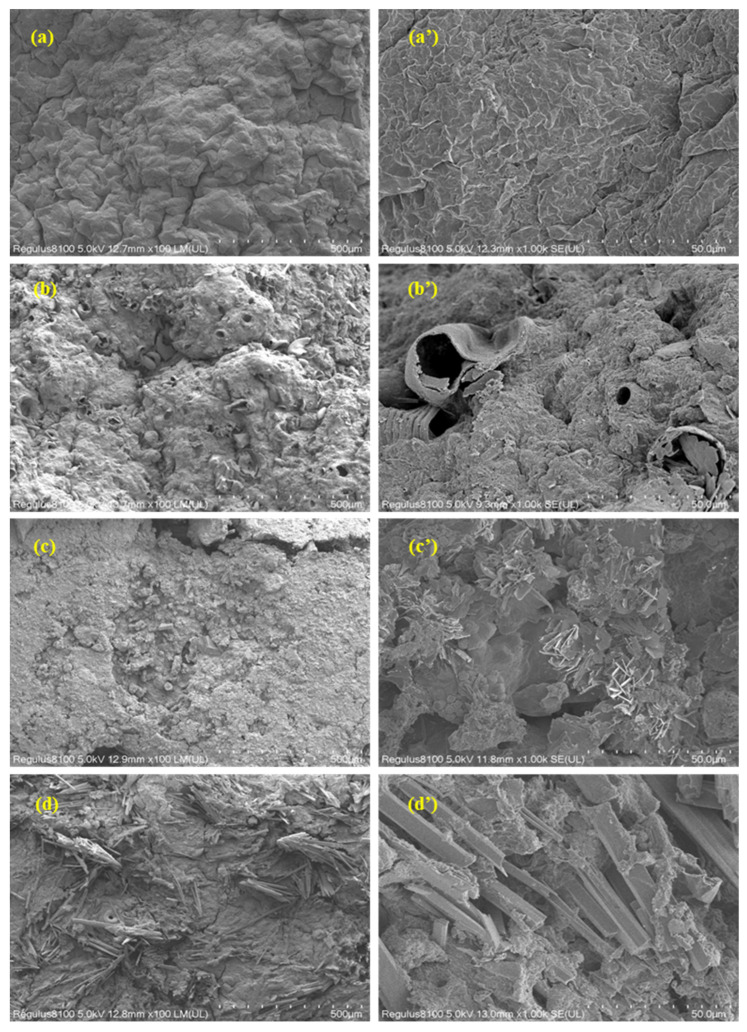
The SEM images of the filter cake after different conditionings: (a) raw sludge at the scale of 500 μm; (a’) raw sludge at the scale of 50 μm; (b) ultrasonic-pretreated sludge at the scale of 500 μm; (b’) ultrasonic-pretreated sludge at the scale of 50 μm; (c) Fe^2+^/PDS-treated sludge at the scale of 500 μm; (c’) Fe^2+^/PDS-treated sludge at the scale of 50 μm; (d) ultrasonic- Fe^2+^/PDS treated sludge at the scale of 500 μm; (d’) ultrasonic-Fe^2+^/PDS treated sludge at the scale of 50 μm.
